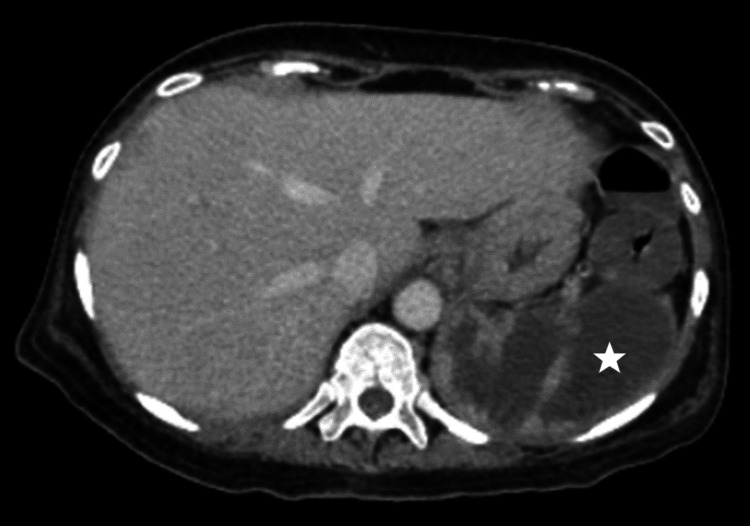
CT abdomen revealing loculated splenic collection (white star).
